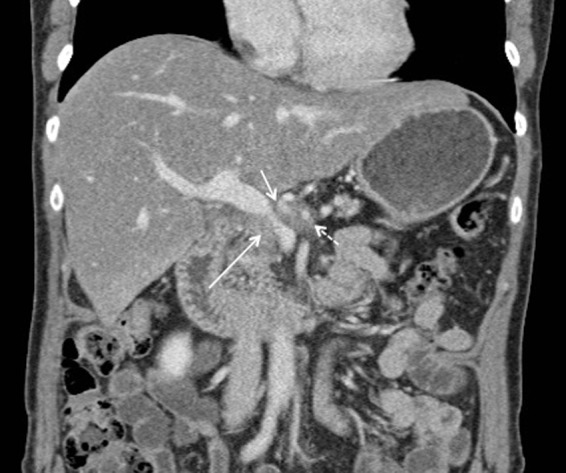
Coronal contrast-enhanced CT showing portal vein–SMV confluence narrowing because of extrinsic tumor compression (long solid arrow). Also seen is proximal common hepatic artery (short solid arrow) and proximal splenic artery (short dashed arrow) encasement, without SMA involvement. SMV, superior mesenteric vein.
